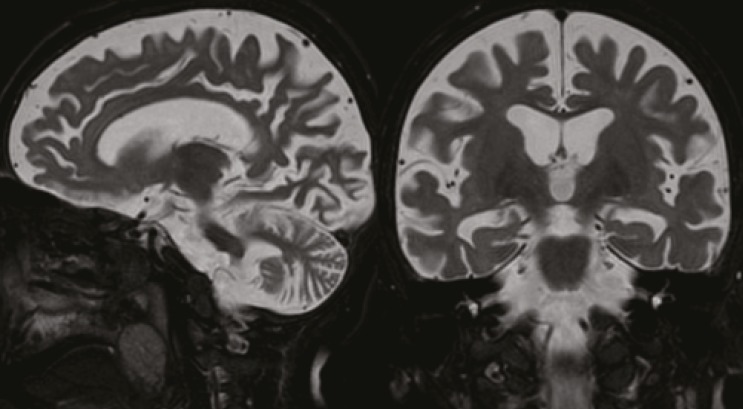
Magnetic resonance tomograms, T2-weighted, of a 5½-year-old child with CLN2 disease, late infantile. Cerebral and cerebellar atrophy is evident
